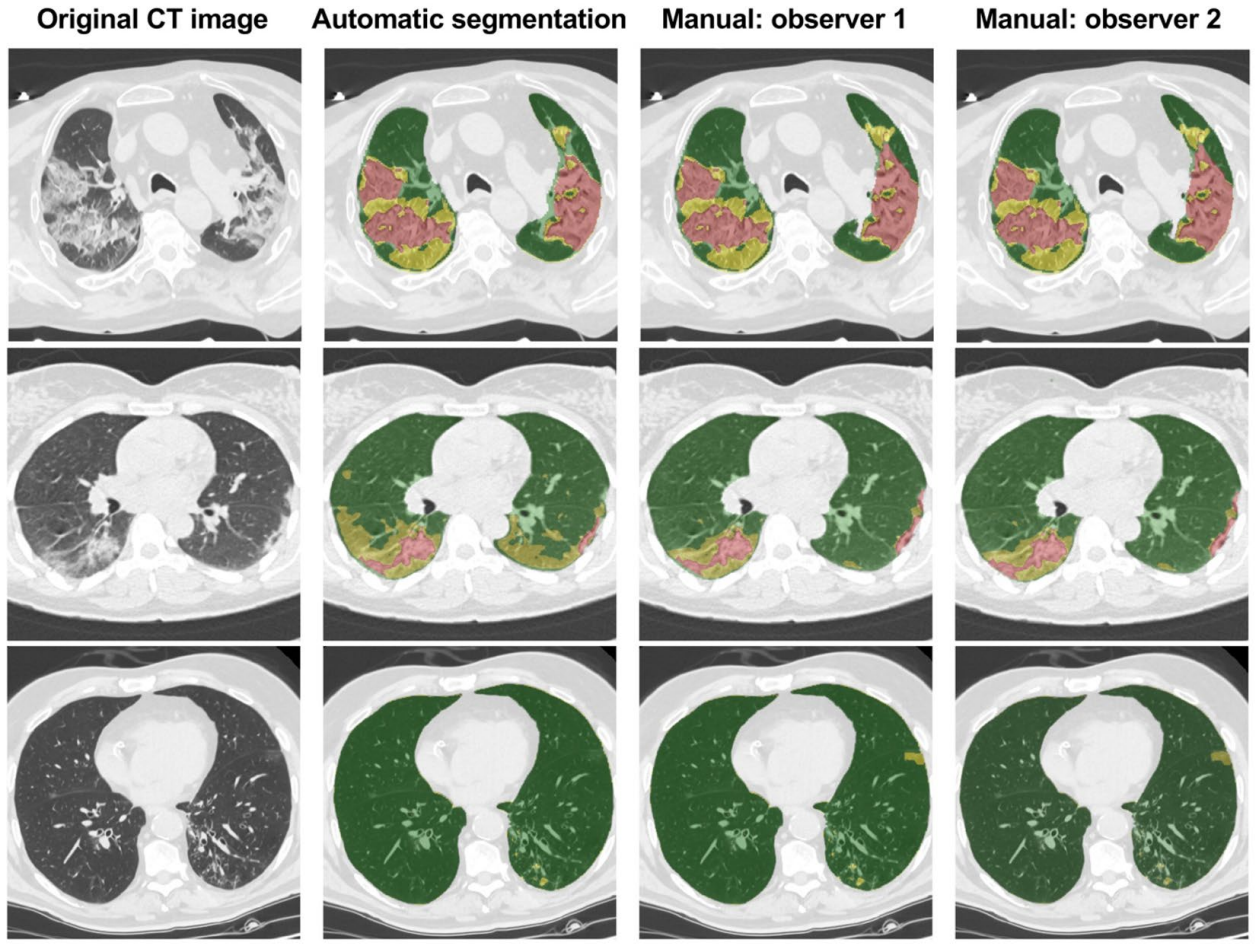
Coronavirus Disease 2019 (COVID-19) lesion subtyping results of three cases with different parenchymal involvement (from top to bottom, 74 year old man, 59 year old woman, 69 year old man, from extensive to little involvement). Nonenhanced CT scans in the axial view (left column) overlaid with the automatic lesion subtyping segmentation (second column) and the corrected scans by the two observers (3rd and 4th columns) are shown. Colors correspond to healthy parenchyma (green), ground glass opacities (yellow) and consolidation (reddish).
